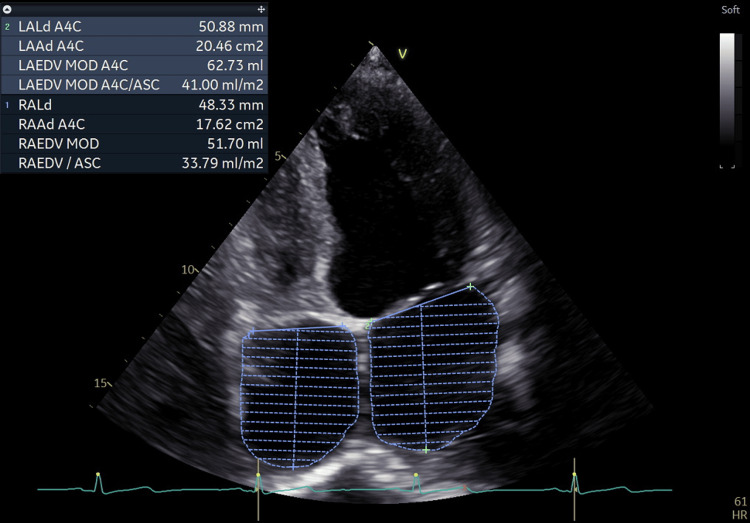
Transthoracic echocardiogram showing the biatrial dilation
